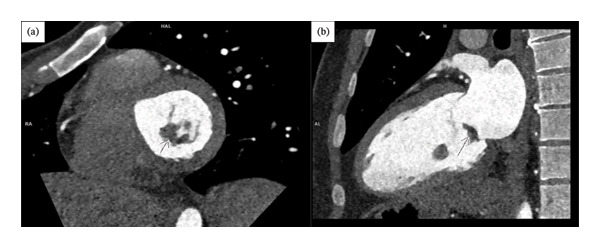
CTA showed a hypodense, mass‐like lesion on the atrial side of the anterior mitral valve leaflet (arrows). ((a) Short axis view at the level of mitral valve and (b) Sagittal view, CTA: computed tomography angiography).
